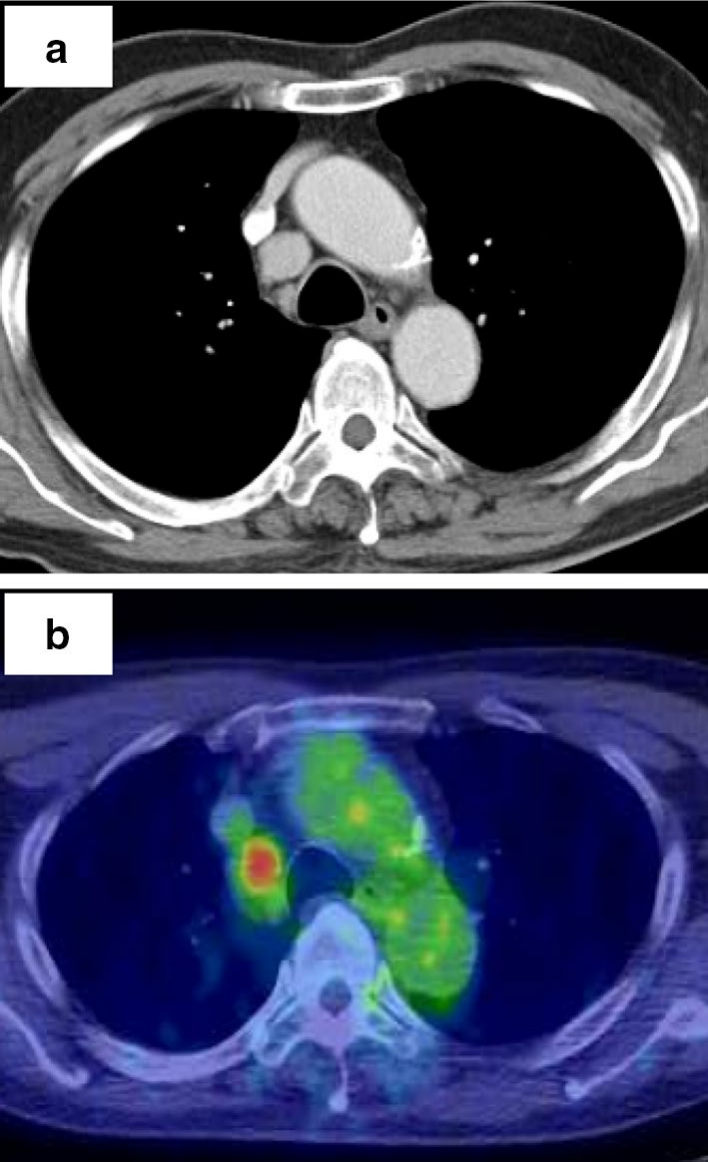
Ectopic thymoma detected in the right side of the paratracheal region by computed tomography (CT) and fluorodeoxyglucose-positron emission tomography (FDG-PET) examination. a Enhanced CT of the chest showed a solitary, well-defined nodule in the right paratracheal region. b The mediastinal tumor showed a marked increase in the accumulation of FDG
